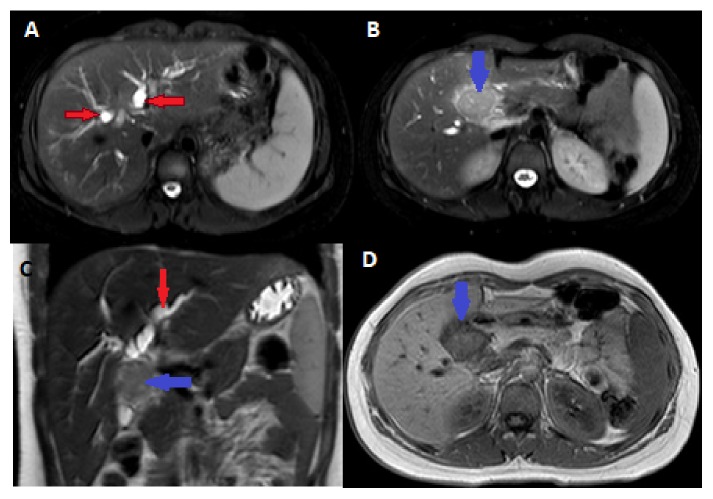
Axial (A, B) and coronal (C) T2-weighted images show a hyperintense mass in the region of the porta hepatis (blue arrow) with a dilated biliary ducts (red arrow). The mass (blue arrow) is hypointense in T1-weighted images (D).
